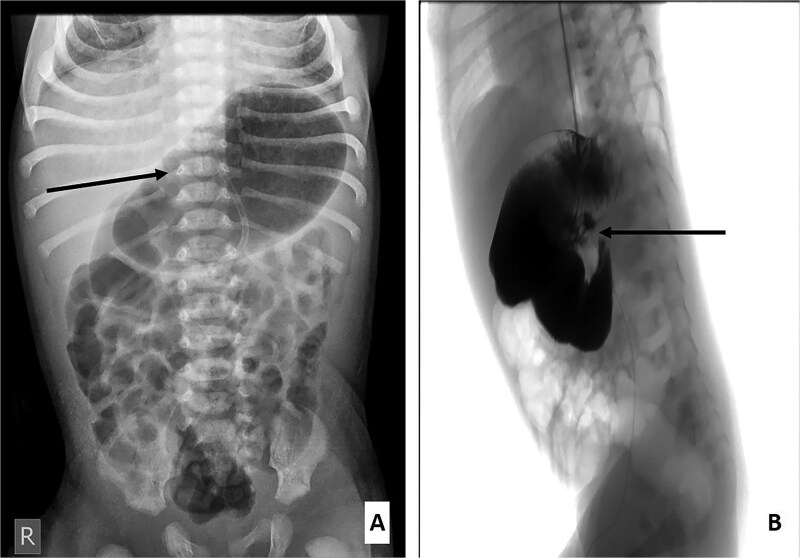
(A) Erect abdominal radiograph showing a markedly distended stomach (arrow) with aeration of the small and large intestines. (B) Contrast study demonstrating a markedly dilated stomach and duodenum with a conical narrowing at the duodenojejunal junction (labeled).
